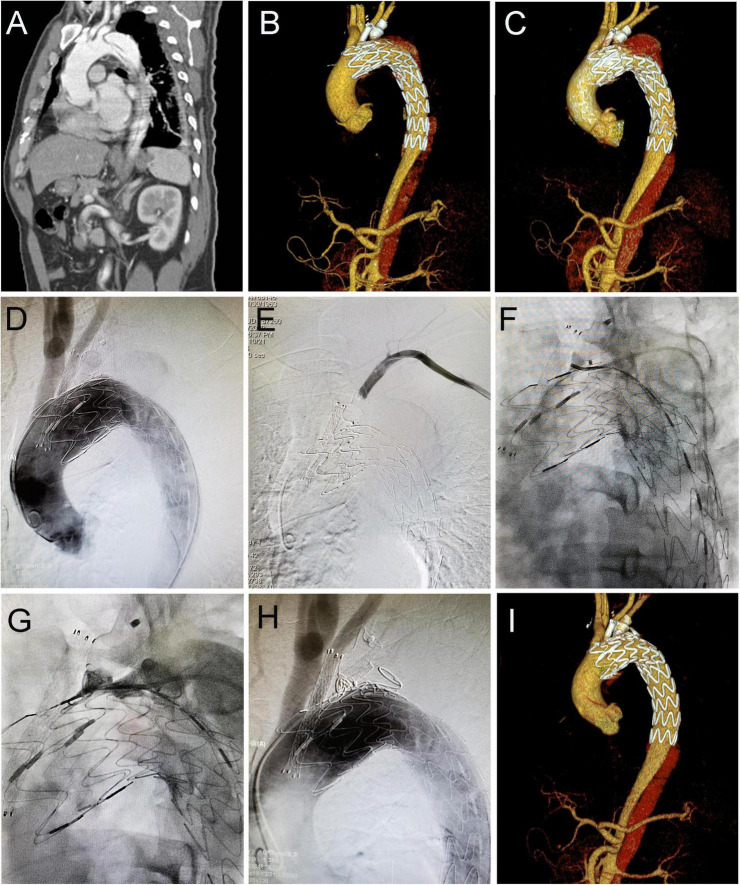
Coil embolization for type IA endoleak 4 years after cTEVAR. Preoperative computed tomography angiography (CTA) showed aortic dissection involving the arch (A). The patient underwent cTEVAR with a chimney stent implanted in LCCA and duct occluder implanted in LSA, and postoperative CTA showed a type IA endoleak (B). The follow-up CTA 4 years later showed the endoleak was significant enlarged (C). With the catheter located in ascending aorta, an angiogram showed contrast could enter the false lumen (D). With the catheter located in LSA, the angiogram showed no contrast entering false lumen (E). With the catheter located in the false lumen, an angiogram showed contrast could enter the aorta (F). After deployment of coils in the false lumen, an angiogram showed contrast could not enter the aorta (G). Post-intervention angiogram (H) and CTA (I) showed the endoleak completely disappeared. cTEVAR, TEVAR with chimney; CTA, computed tomography angiography; LCCA, left common carotid artery; LSA, left subclavian artery; aRSA, aberrant right subclavian artery.
